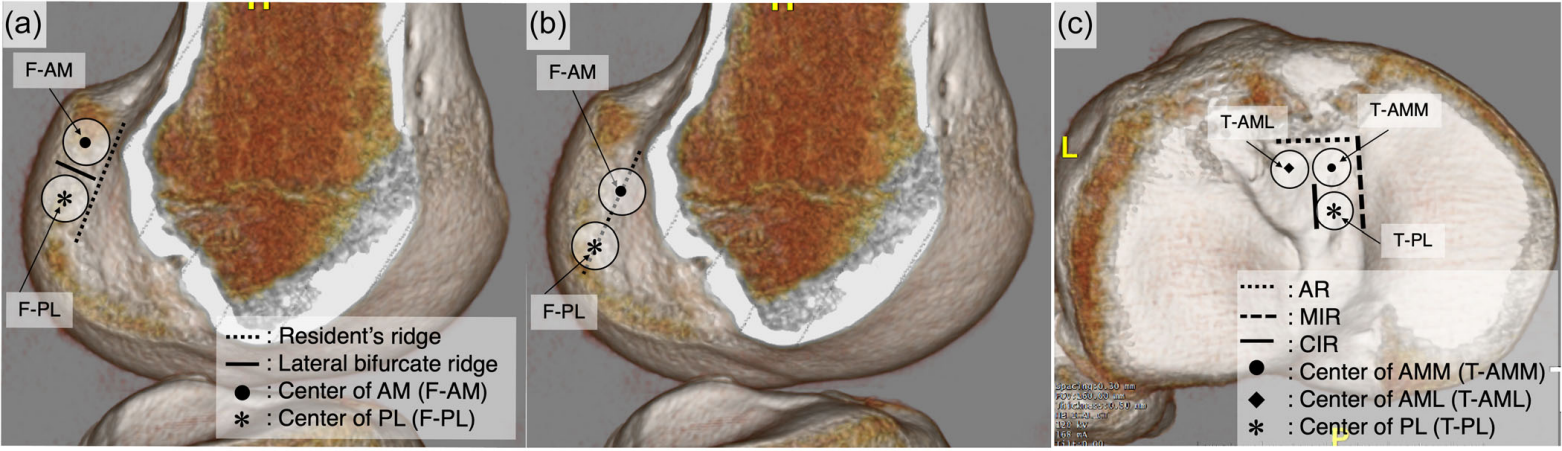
The positions of the femoral and tibial attachments of each ACL fibre bundle on 3D CT. (a) The femoral attachment site placed behind the resident's ridge (RR) (7‐mm‐diameter circle each). (b) The femoral attachment site placed on the RR (7‐mm‐diameter circle each). (c) The tibial attachment site (5‐mm‐diameter circle each). 3D, three‐dimensional; ACL, anterior cruciate ligament; AM, anteromedial bundle; AML, anteromedial bundle lateral part; AMM, anteromedial bundle medial part; AR, anterior ridge; CIR, central intercondylar ridge; F‐AM, centre of AM attachment on the femur; F‐PL, centre of PL attachment on the femur; MIR, medial intercondylar ridge; PL, posterolateral bundle; T‐AML, centre of AML attachment on the tibia; T‐AMM, centre of AMM attachment on the tibia; T‐PL, centre of PL attachment on the tibia.
